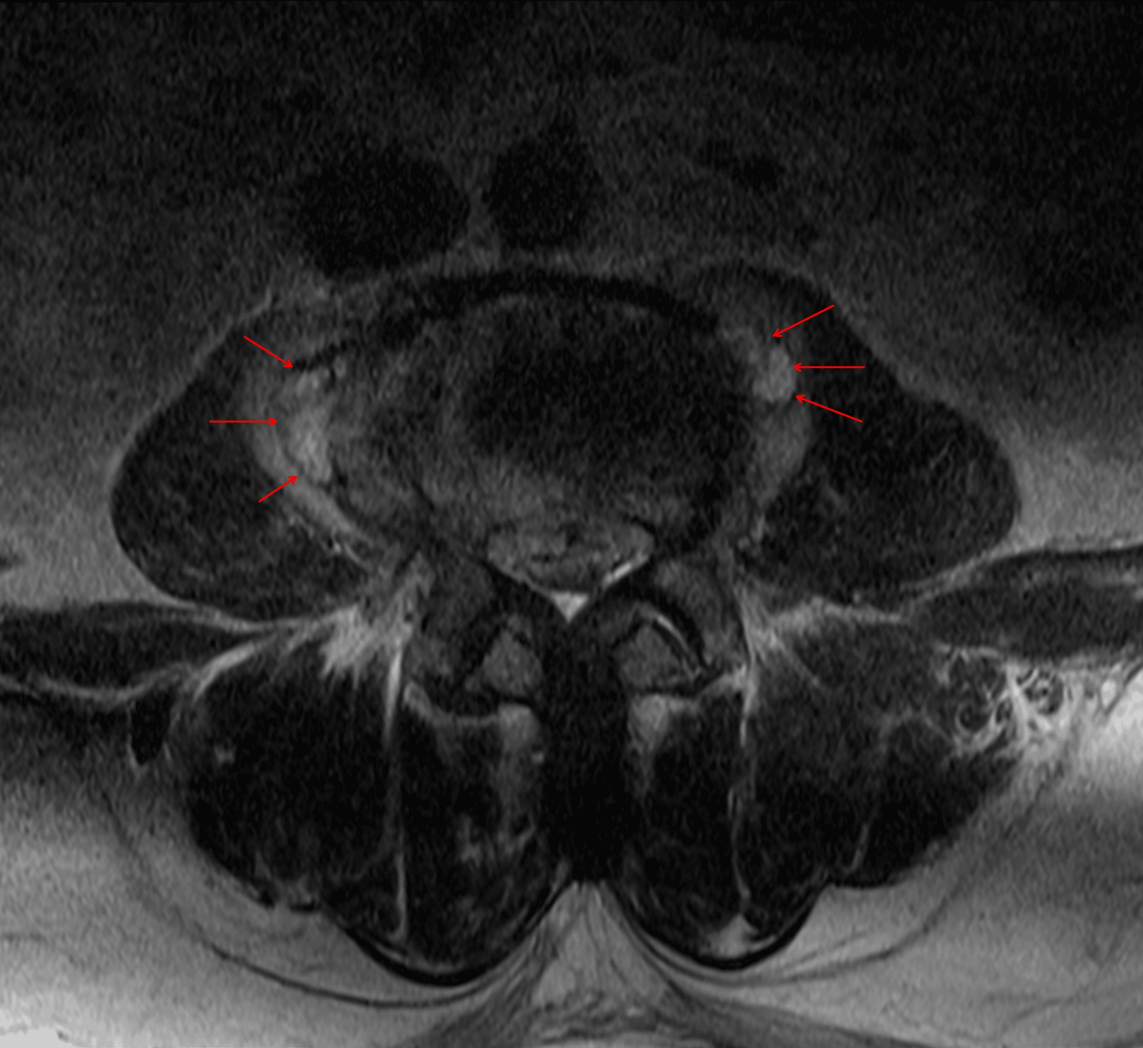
Spine axial magnetic resonance imaging (MRI) was obtained in the second week of hospitalization. A T2-weighted image shows several newly noted abscess pockets in bilateral psoas muscles at L4 levels.
